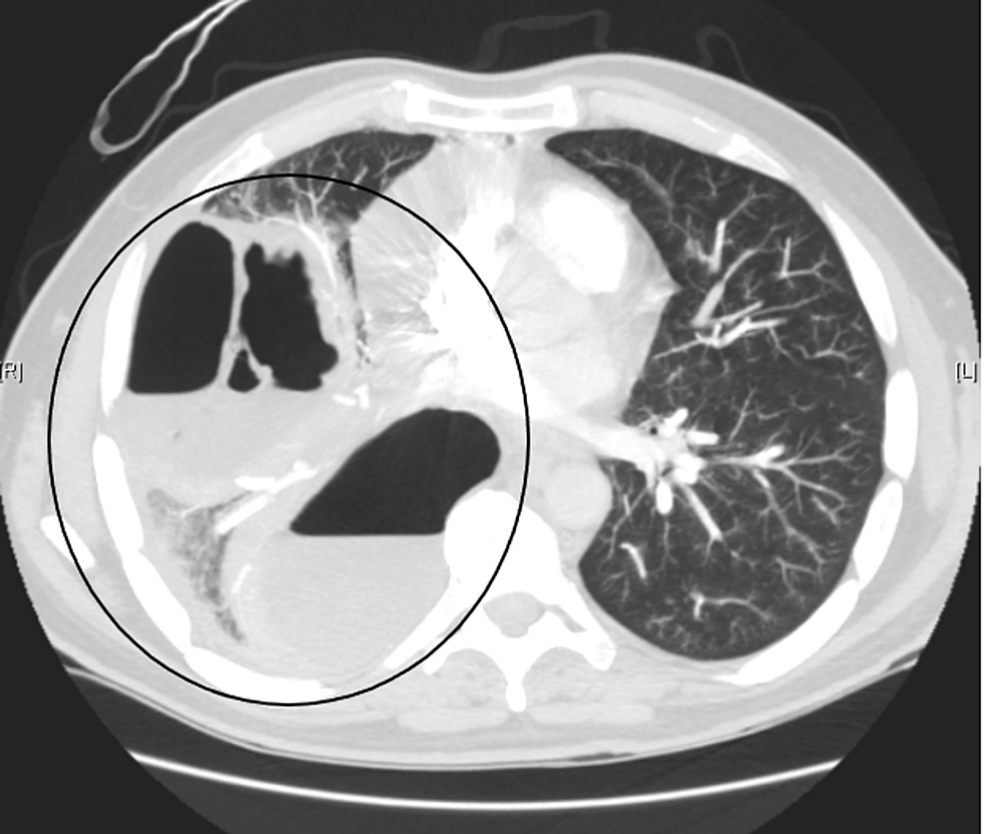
CT of the chest showing significant interval worsening of cavitary right middle lobe lesion, now demonstrating an internal air-fluid levels and gas-filled septations and measuring up to 18.6 cm. This likely represents evolution of lung necrosis with probable superimposed infection. New small hydropneumothorax along the convexity of the right upper lung is also noted.
